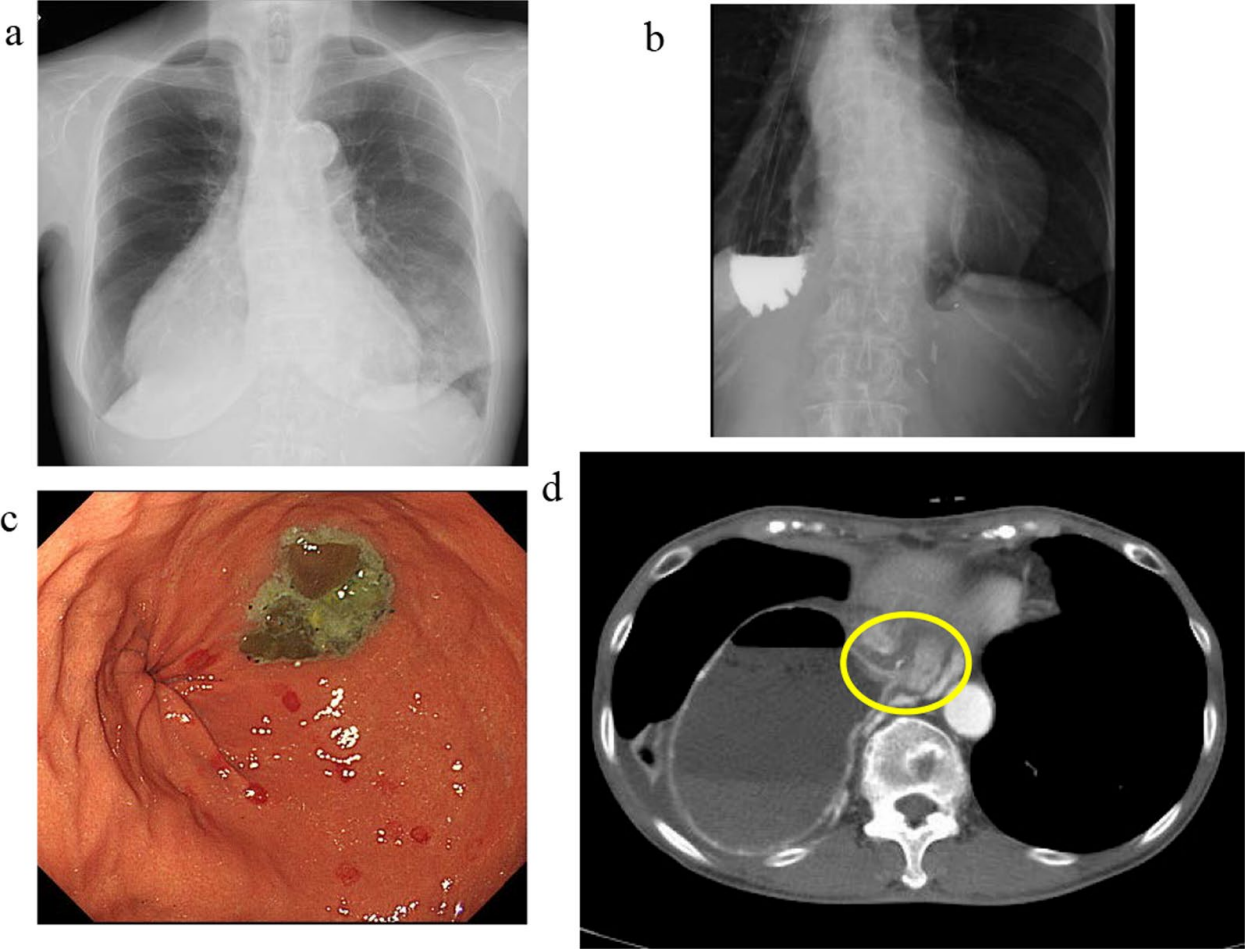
Examination findings when the patient complained of vomiting. a A dilated gastric tube is visible in the thoracic cavity on chest X-ray. b Contrast medium is retained in the thoracic part of the gastric tube on fluoroscopy. c On upper gastrointestinal endoscopy, deflection and torsion of the gastric tube is visible at the site of the thoracoabdominal transition. d On CT, a severely dilated gastric tube is visible in the thoracic cavity with a stricture (yellow circle). *CT* computed tomography
